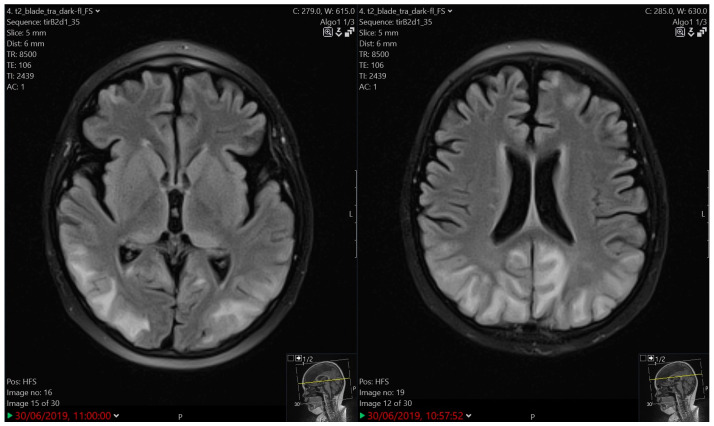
PRES. An MRI finding of subcortical oedema of white matter in occipital and parietal lobes and the posterior segments of the temporal lobes. Smaller oedemas are also observed in the frontal regions.
